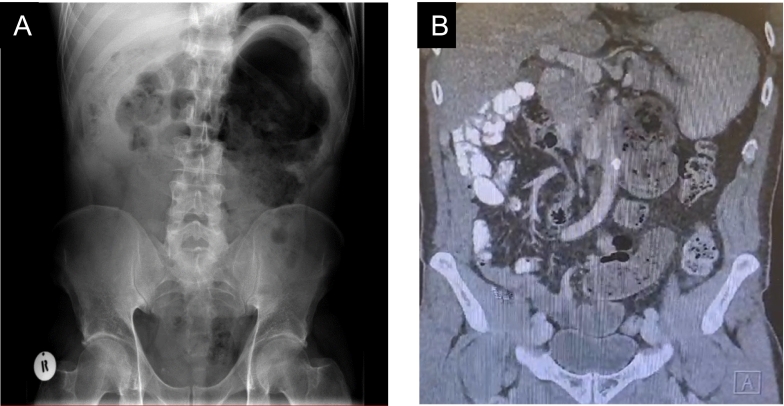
Abdominal Xray and CT abdomen pelvis of Case 1.
